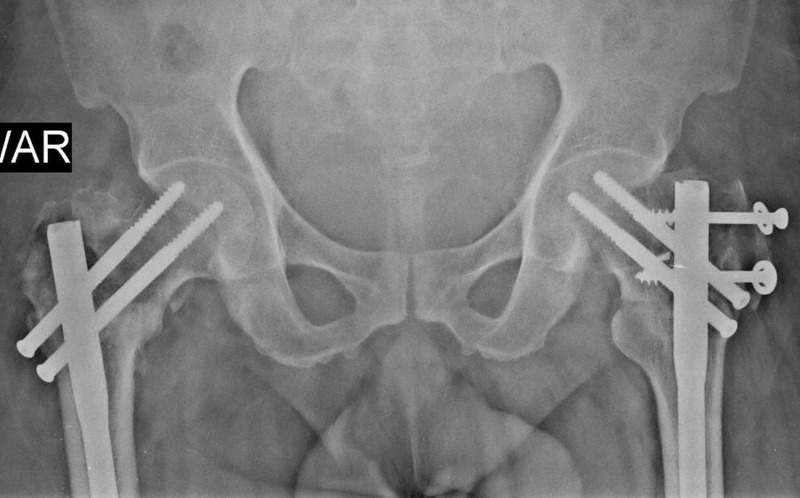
13 months post-operative x-ray of the pelvis showing union of both the fractures
